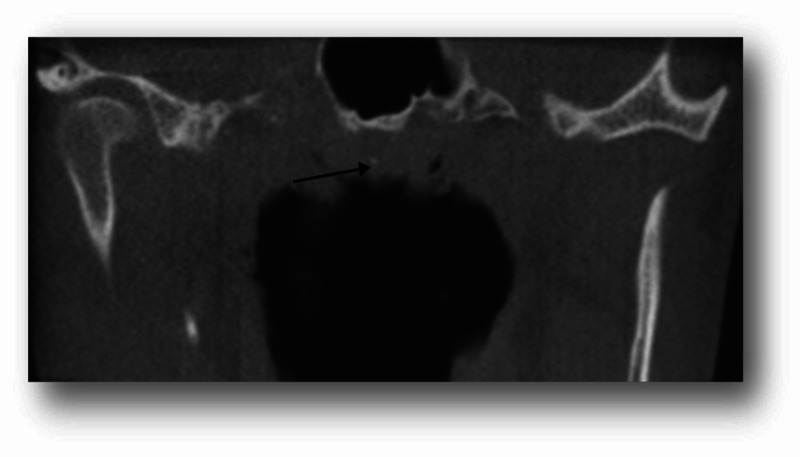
Adenoid calcification in a 43-year-old male A 43-year-old male patient presented for dental treatment (asymptomatic). A frontal cut of the CBCT, FOV: 6 cm (maxilla) showed a single adenoid calcification (black arrow). CBCT: cone-beam computed tomography; FOV: field of view
